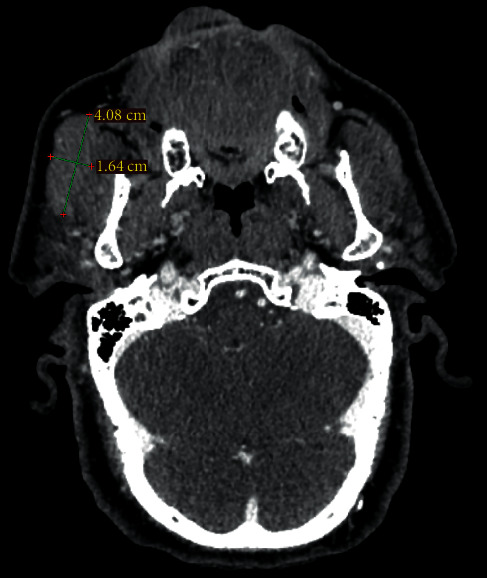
Computed tomography showing a buccal space mass with irregular margins and invasion of the neighboring masseter muscle.
